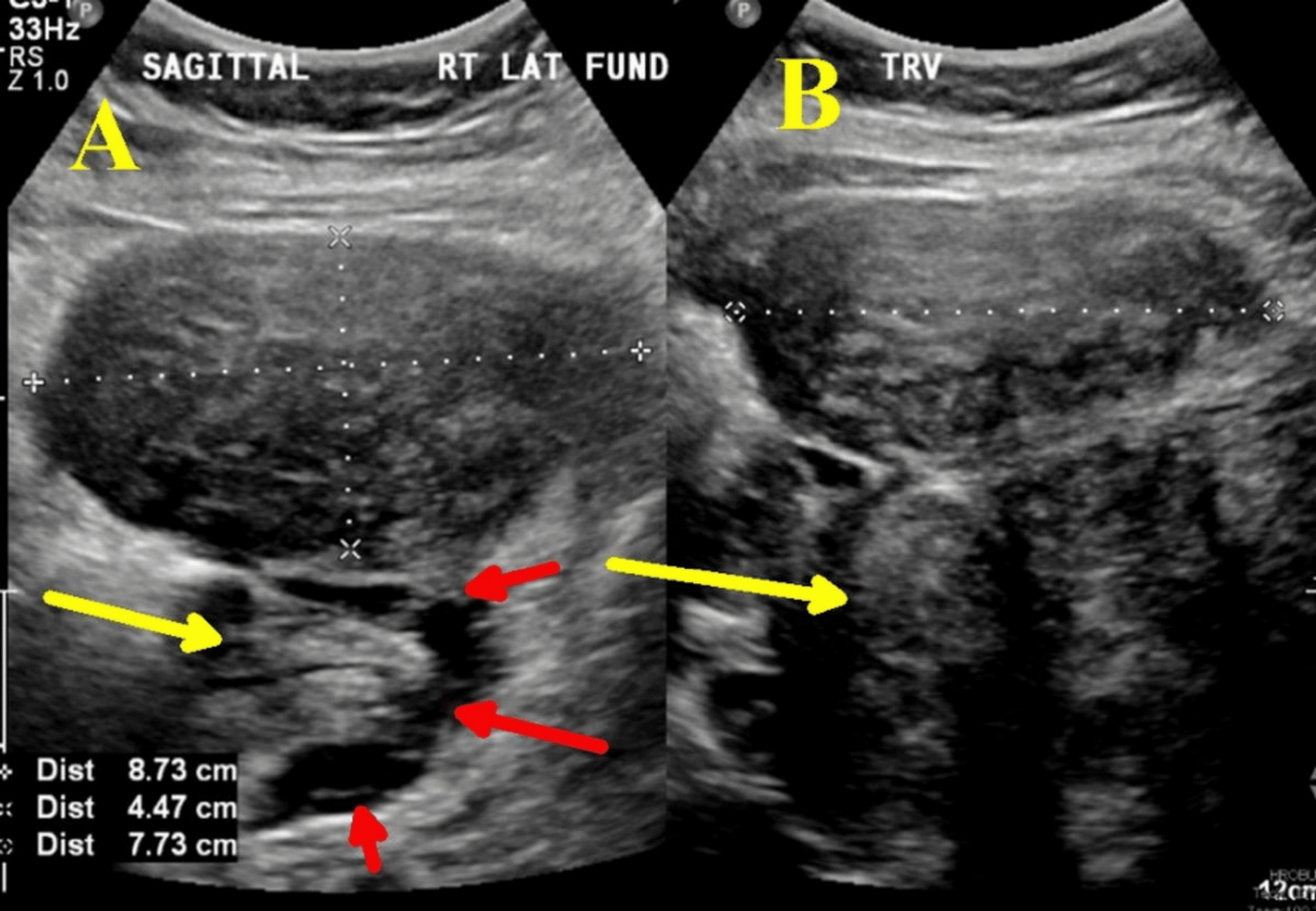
Obstetric ultrasound on admission at 23 weeks and 5 days Image A is a sagittal view, and image B is a transverse view. The yellow arrows on A and B show the fetus; the red arrows in image A show the amniotic fluid, and one of her myomas is between the hashmarks in images A and B, measuring 8.73 cm x 4.47 cm x 7.73 cm.
